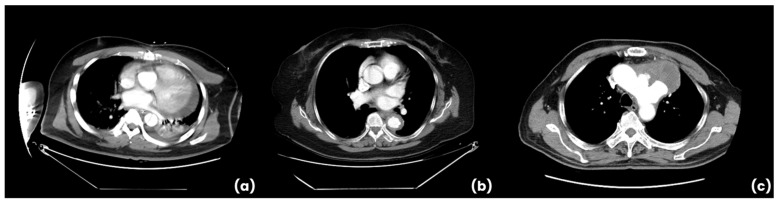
Representative CTA images from the study dataset demonstrating typical manifestations of AAS subtypes. (a) Aortic dissection (AD). (b) Intramural hematoma (IMH). (c) Penetrating atherosclerotic ulcer (PAU).
